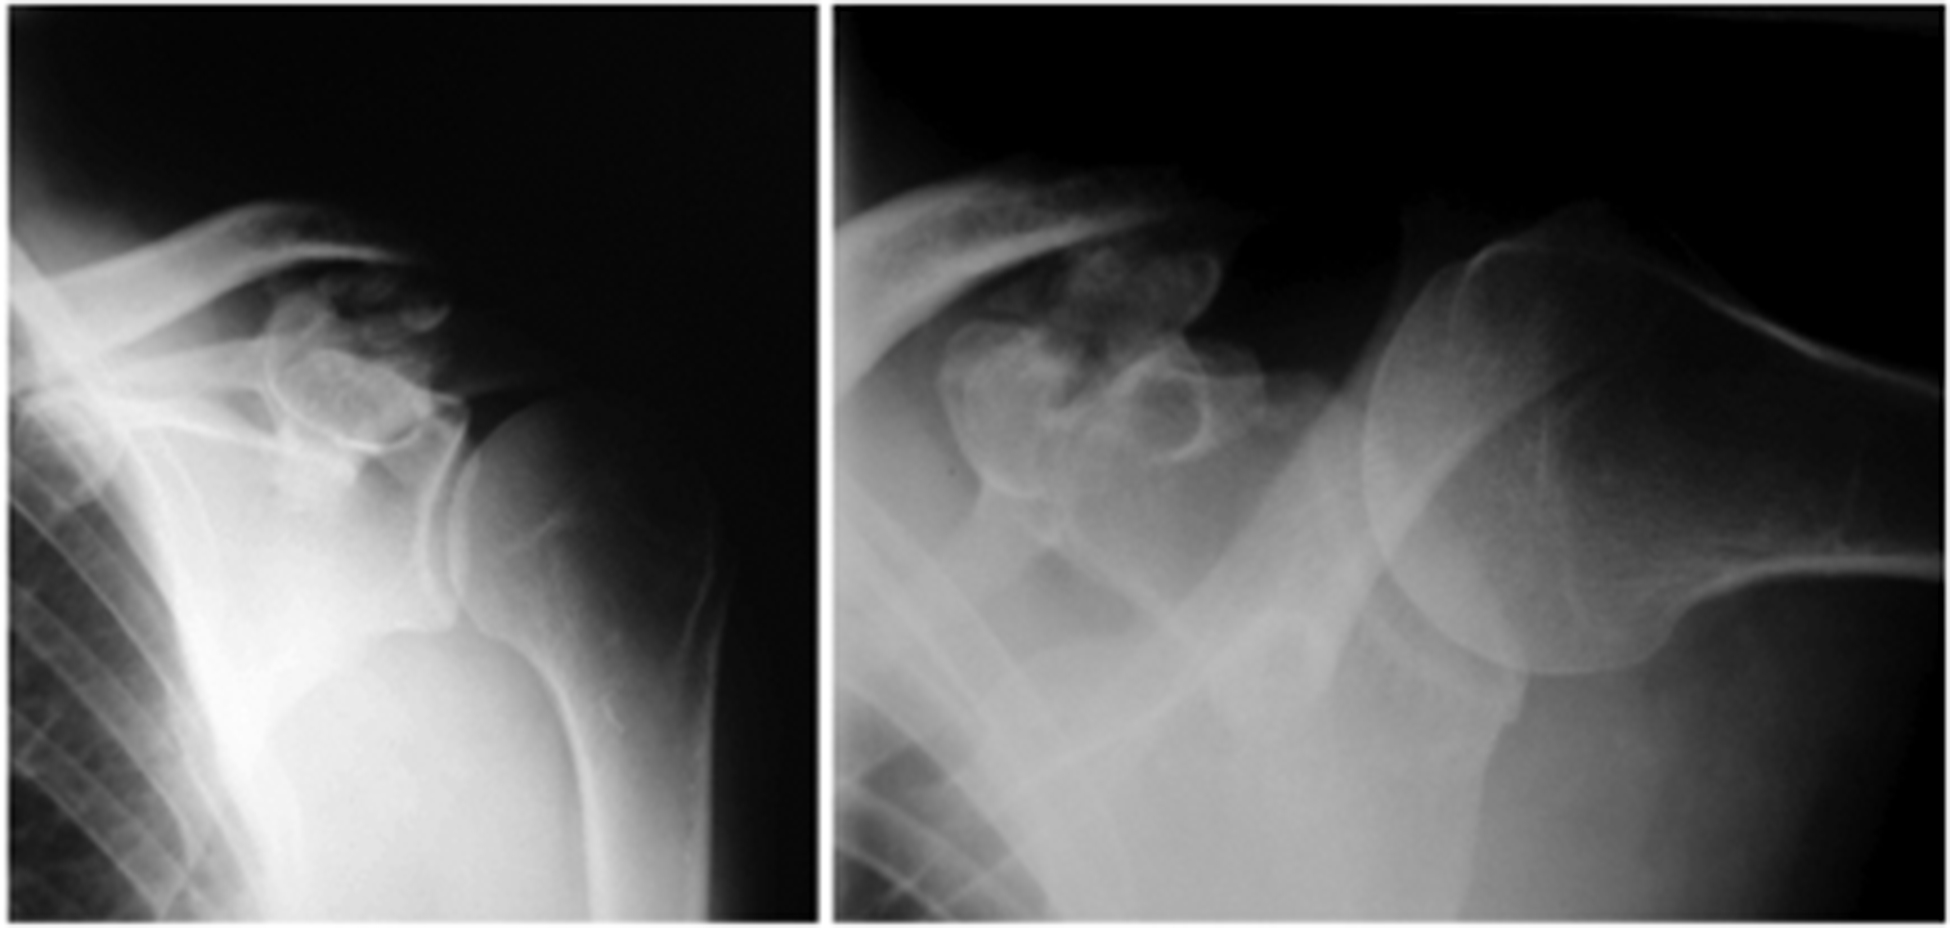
X-Ray of the left shoulder a.p. view a. and lateral view; b. shows osteoarthritis of a coracoclavicular joint with joint space narrowing, osteophytes and several loose bodies consistent (in the ap view).
